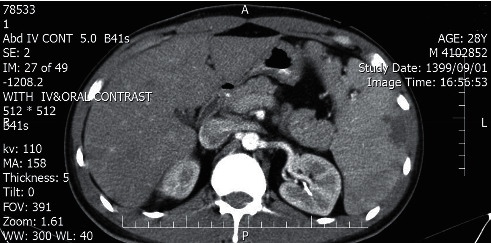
Abdominal CT scan with IV contrast shows splenic enlargement with a wedge-shaped, low-density defect in the peripheral area of the spleen in favor of splenic infarction.
